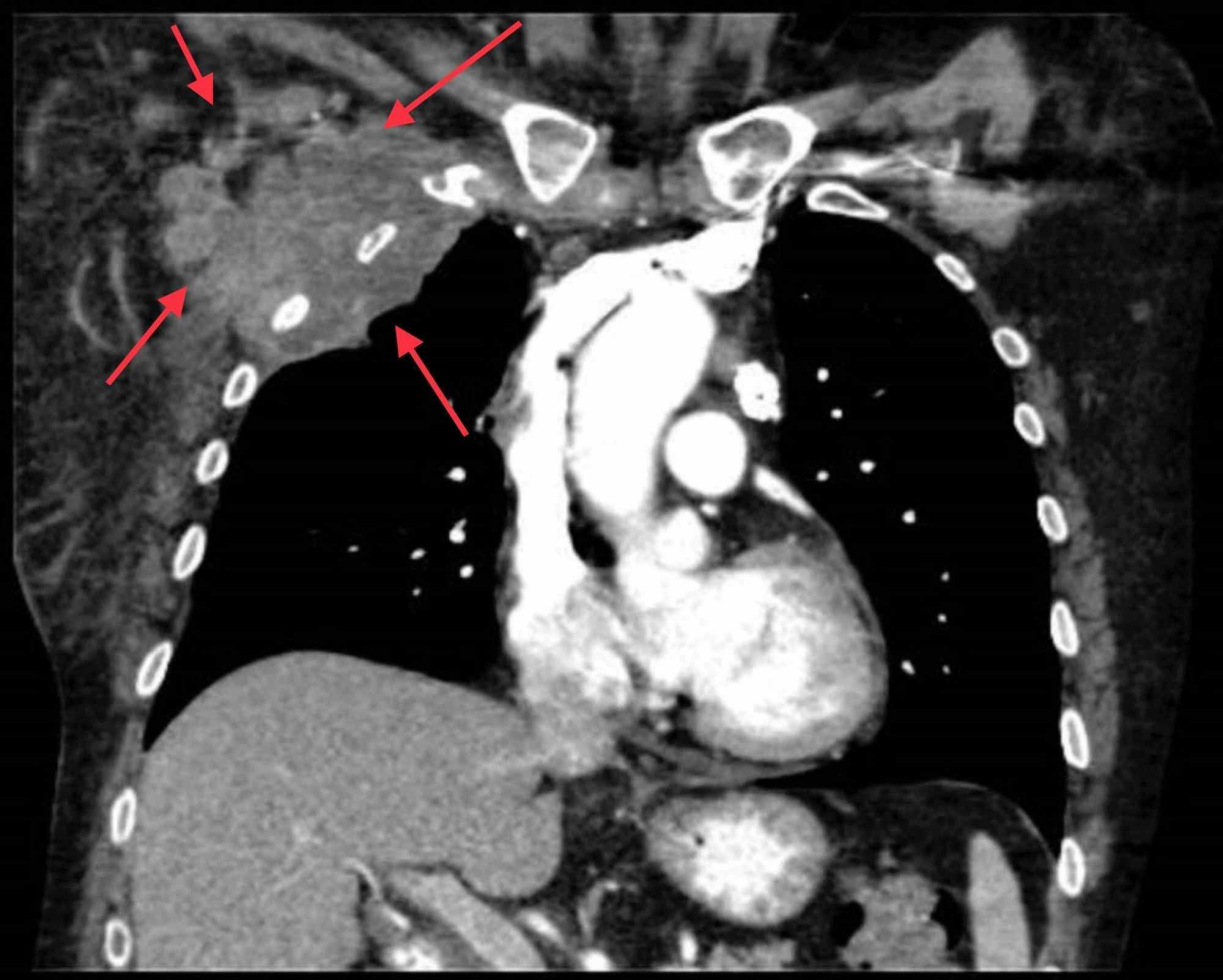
Coronal CT scan The arrows show large mass along the right chest wall measuring 8.6 x 8 cm with adjacent conglomerate adenopathy. There is associated destruction of right ribs 2 and 3 with pathologic fractures CT: computed tomography
